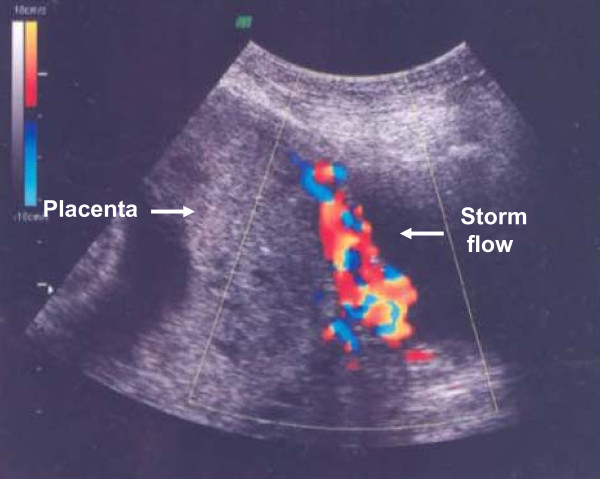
Ultrasonography findings at gestational week 29 consistent with placenta accreta.
